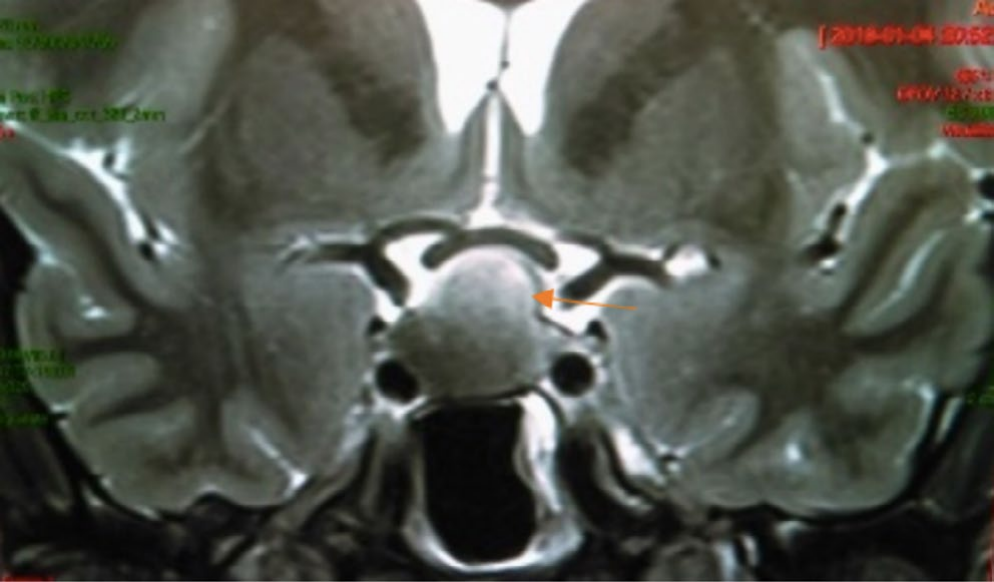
T2‐weighted MRI of the sellar region showing an heterogeneous, partially cystic pituitary lesion with evidence of recent hemorrhage, measuring 18.7 × 13.5 × 18.7 mm (arrow).
